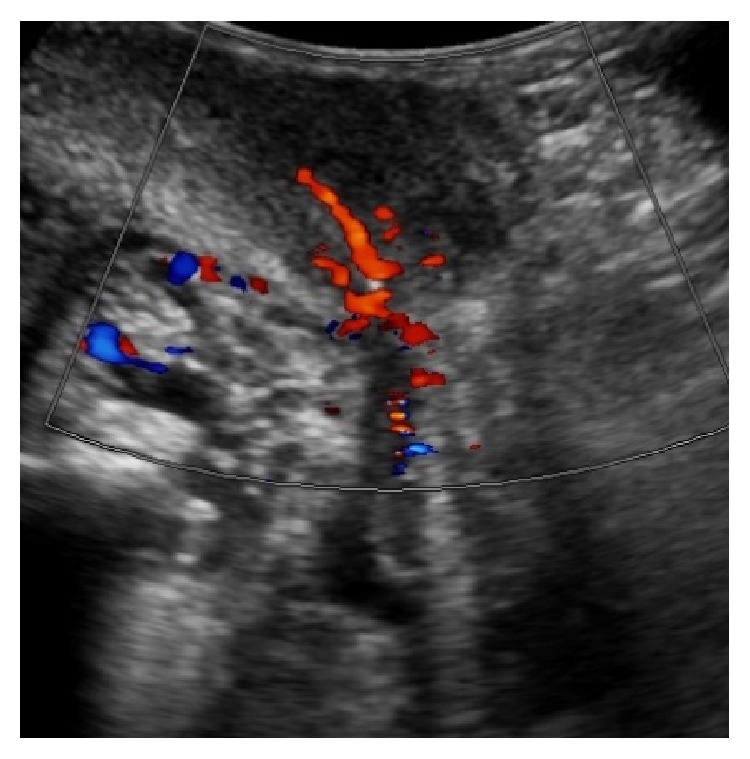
Urethral bulb and prostate in a patient rendered incontinent after transurethral resection of the prostate. Duplex Doppler showing blood flow within proximal bulb and prostate.
